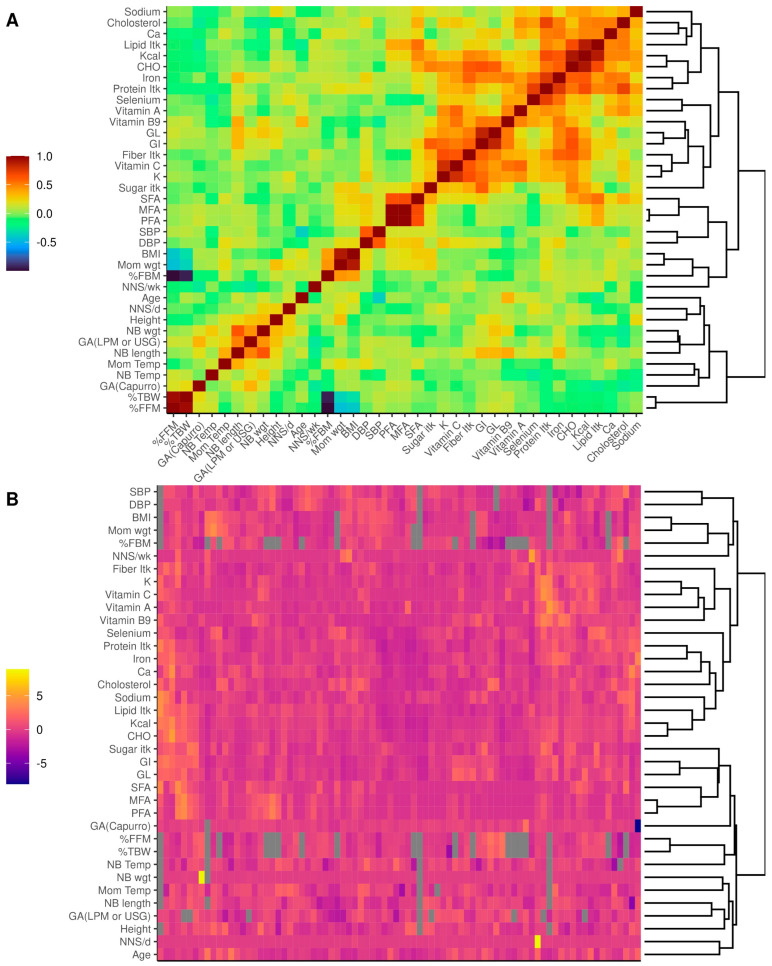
(A) Spearman correlation heatmap of continuous metadata measured in the study. Variable names are indicated in the X and Y axes, the right dendrogram shows the optimal leaf ordering (OLO) of metadata, and the left color bar indicates a strong positive correlation (1), no correlation (0), or strong negative correlation (−1). (B) Distribution heatmap of continuous metadata measured in the study. Variable names are indicated in the y−axis, the x side of the matrix represents each sample included in the study, the right dendrogram shows the OLO of samples and metadata, and the left color bar indicates a centered t−distributions of the metadata. Ca: calcium; Itk: intake; CHO: carbohydrates; GL: glycemic load; GI: glycemic index; SFA: saturated fatty acids; MFA: monounsaturated fatty acids; PFA: polyunsaturated fatty acids; DBP: diastolic blood pressure; SBP: systolic blood pressure; BMI: body mass index; Mom: mother; wgt: weight; % FBM: percentage of fat body mass; NNS: non−nutritive sweeteners; wk: week; d: day; NB: newborn; Temp: body temperature in °C; GA: gestational age; LMP: last menstrual period; USG: ultrasonography; % TBW: percentage of total body water; % FFM: percentage of lean body mass.
